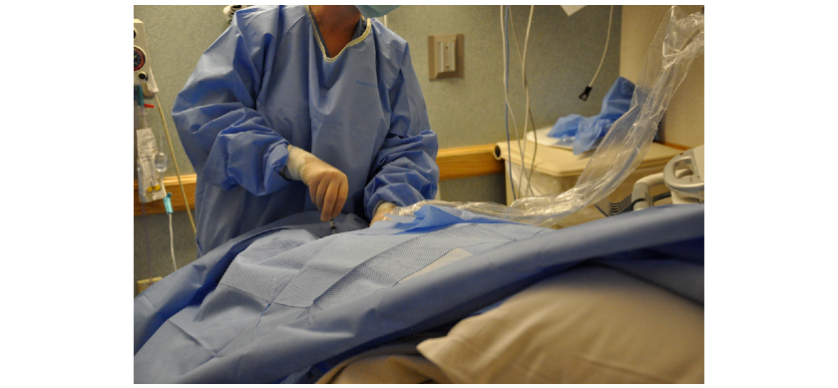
Ultrasound monitor position requires physicians to look away from the access site.
